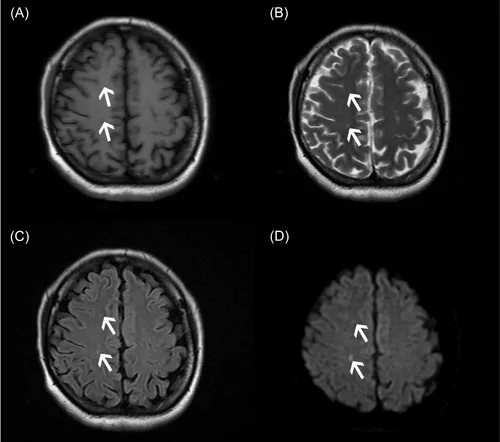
Nodular, slightly elongated T1 and T2 signal intensities are observed in the right semioval center and frontal lobe white matter (A, B, white arrows). There is slight hyperintensity on FLAIR imaging (C, white arrows), and diffusion‐weighted imaging (DWI) shows high signal intensity (D, white arrows).
